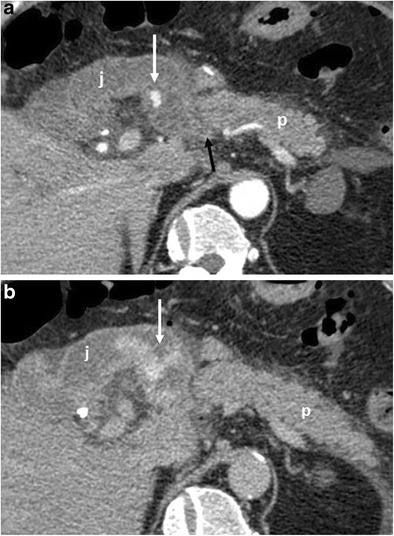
a, b Axial CT curvilinear reconstructions show an active extravasation in the arterial phase (white arrow in a) within the lumen of the jejunal anastomotic loop (j). Bleeding becomes more evident in the late phase (white arrow in b). The pancreatic stump is seen (p)
